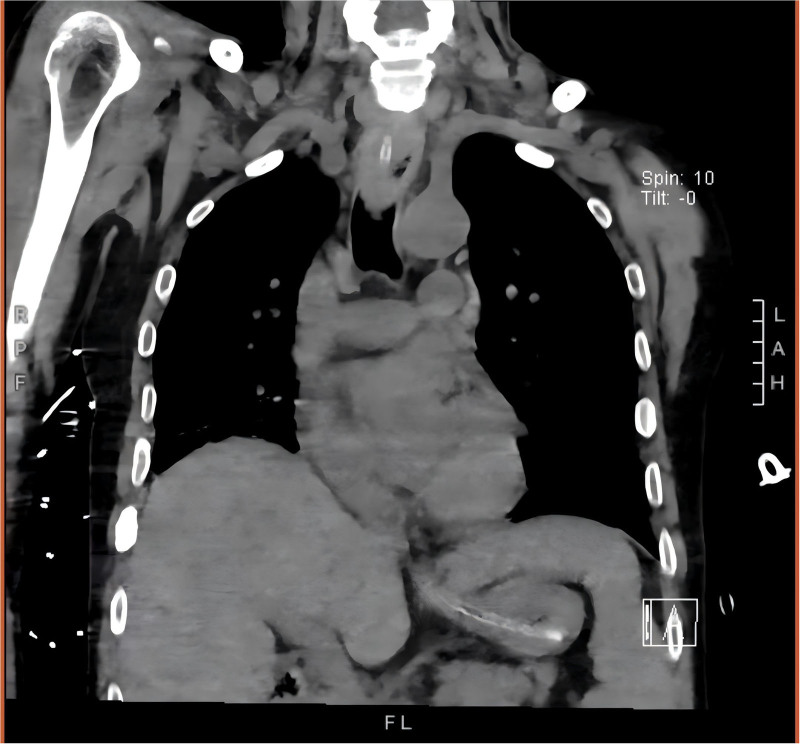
Abdominal CT: the tip of the nasogastric tube is positioned in the stomach. CT = computed tomography.
